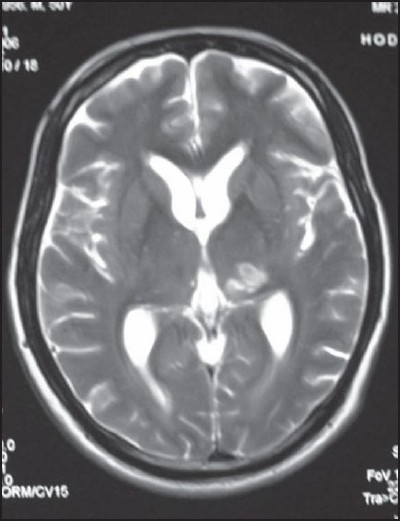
MRI brain, axial view, showing T2 hyperintensity in the left thalamus that is extending into the corona radiata
